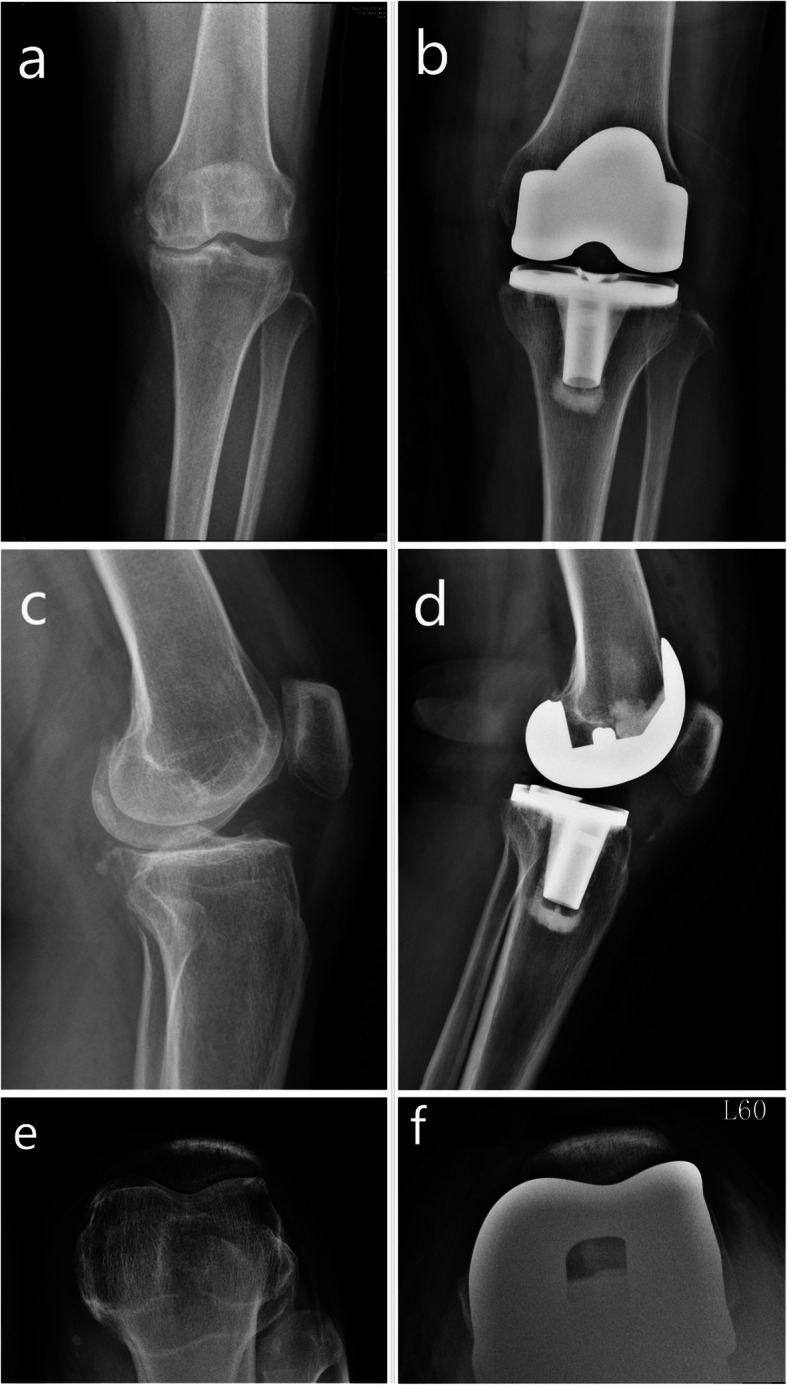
Typical case of posterior-stabilized prosthesis. a Anteroposterior position before surgery in patients with posterior-stabilized (PS) prosthesis. b Anteroposterior position after surgery in patients with PS prosthesis. c Lateral position of the knee joint before surgery in patients with PS prosthesis. d Lateral position of the knee joint after surgery in patients with PS prosthesis. e Patella axial radiograph (60°) before surgery in patients with PS prosthesis. f Patella axial radiograph (60°) after surgery in patients with PS prosthesis
